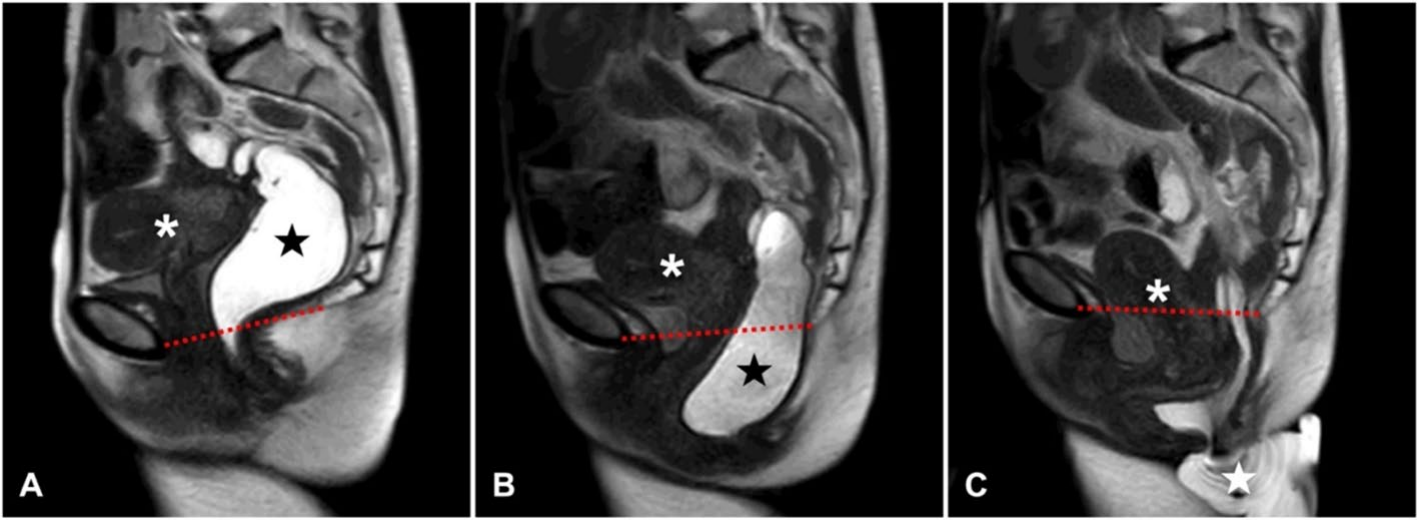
Magnetic resonance image defecography (MRI-D) in sagittal view of a female pelvis with obstructed defecation syndrome (ODS) and pelvic organ prolapse (POP) before surgery. The red dotted arrow marks the pubo-coccygeal line. The white asterisk marks the uterus, the black star marks the filled rectum (ultrasound gel), and the white star marks the excreted ultrasound gel. Figure 2A shows the position at rest, 2B shows the defecation process and descending of the rectum and uterus, and 2C shows the excreted ultrasound gel
